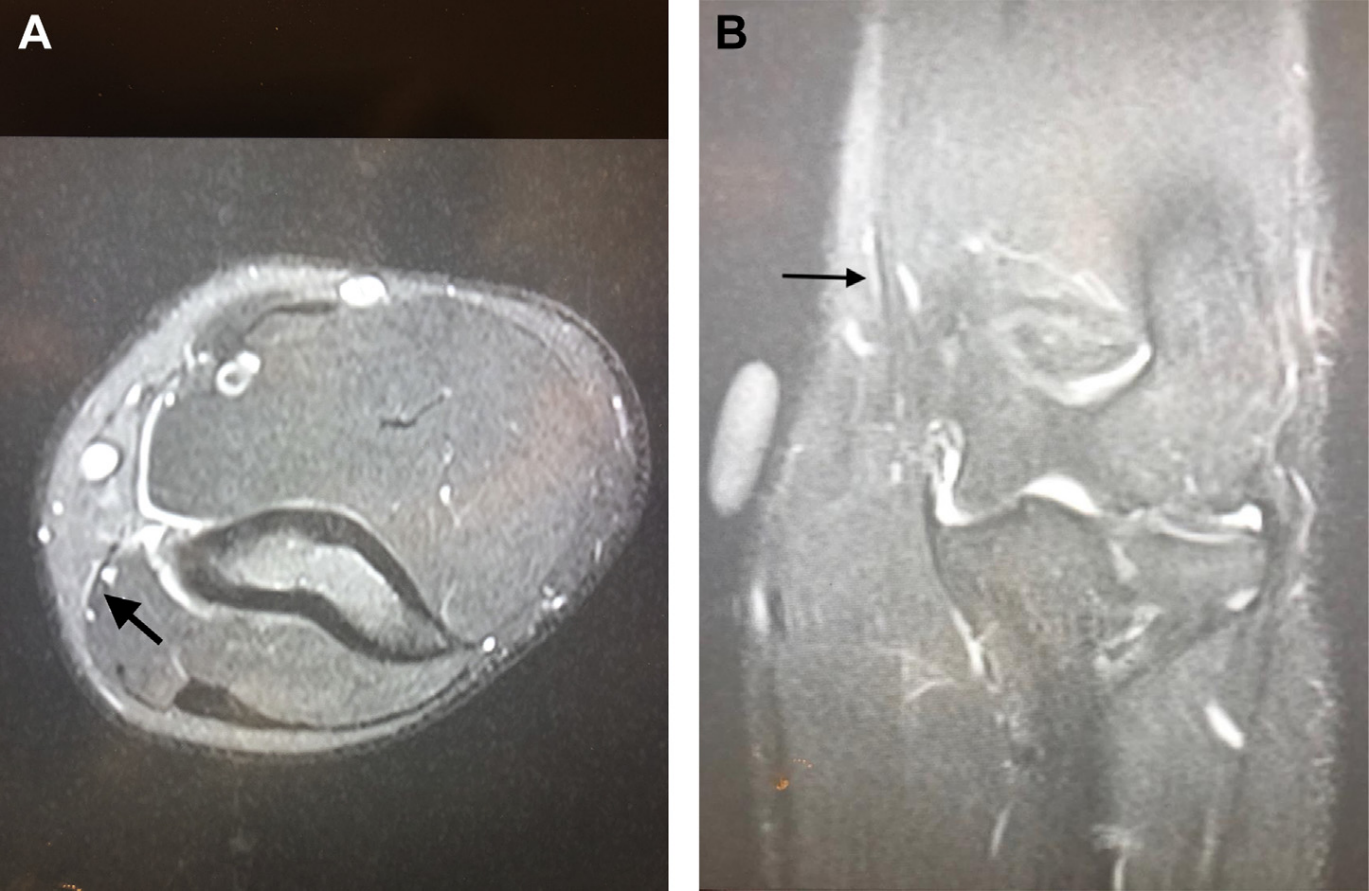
Magnetic resonance imaging scan of the left elbow demonstrates ulnar nerve abutment with a branch of the superior ulnar collateral artery. (A) Axial; (B) Coronal.
